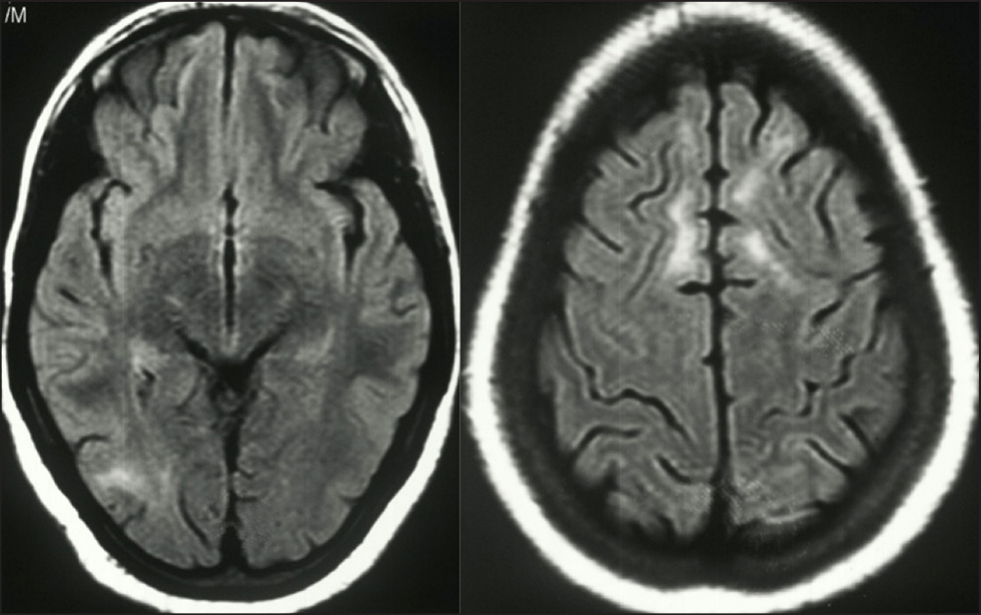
The brain MRI reveals white matter hyperintensities on T2 FLAIR imaging, primarily affecting the right occipital region, bilateral high frontal regions, and additional areas in the left frontal region.
